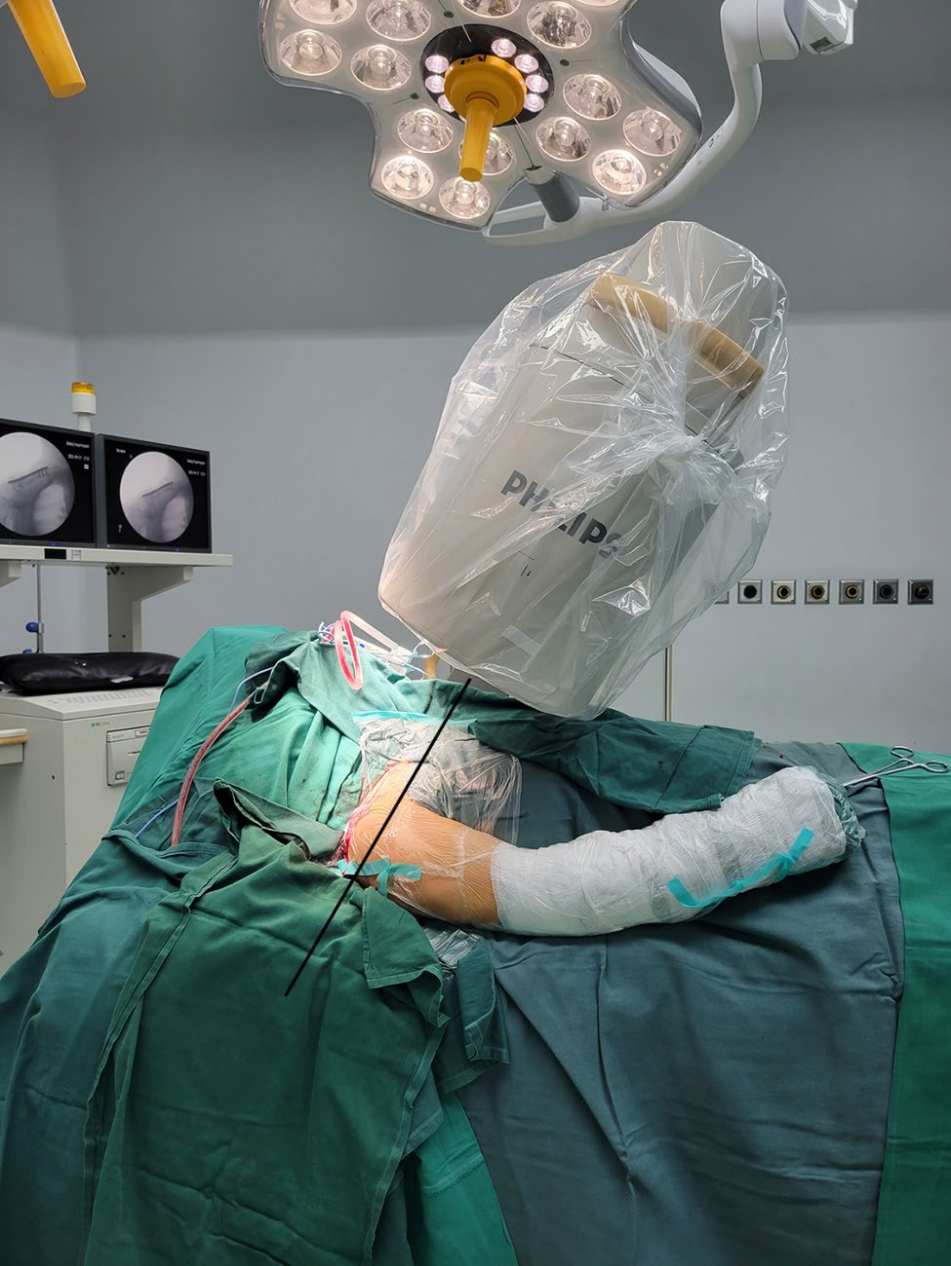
Clinical photograph: PA 25° cephalic skyline fluoroscopic projection of the clavicle with the patient in the supine position The X-ray beam (indicated with a black line) was centered on the middle point of the clavicle
